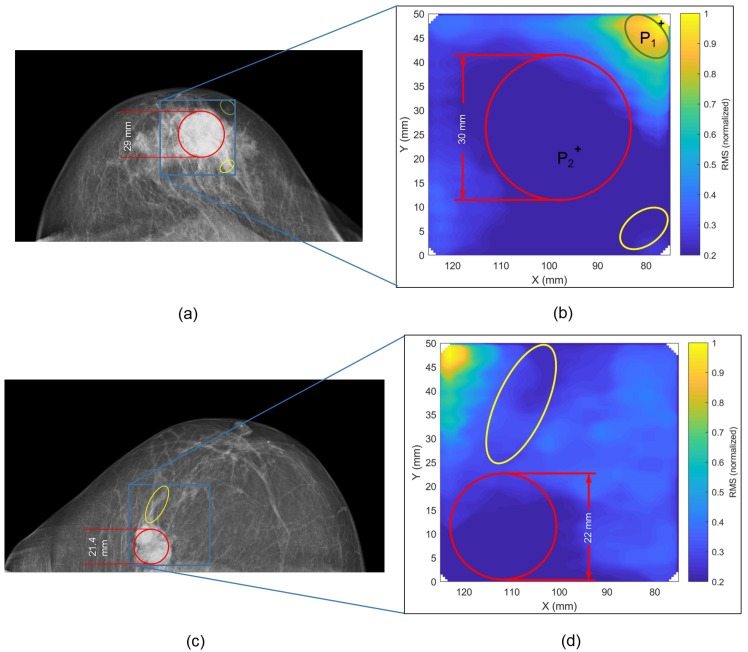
Comparison between X-ray image and microwave image for patient A (a,b) and patient B (c,d). The RMS was normalized by the maximum RMS of the scanned region of the present patient. The lowest intensity values occur in the area of the tumor location given by stronger attenuation of cancerous tissue. The final thickness of the compressed breast during microwave examination is very similar for patients A and B, i.e., 4.4 cm for patient A and 4.3 cm for patient B. During X-ray examination, the breast was slightly more compressed, i.e., 4.1 cm for patient A and 3.7 cm for patient B.
